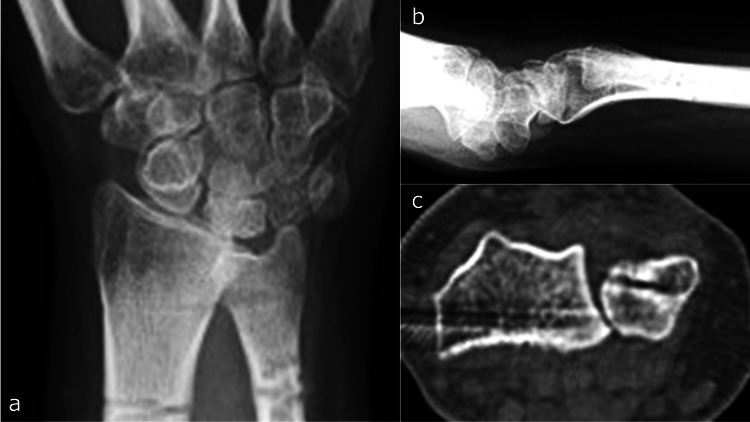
Imaging six months post-injury. a: X-ray anteroposterior view. b: X-ray lateral view. c: Computed tomography axial view.
